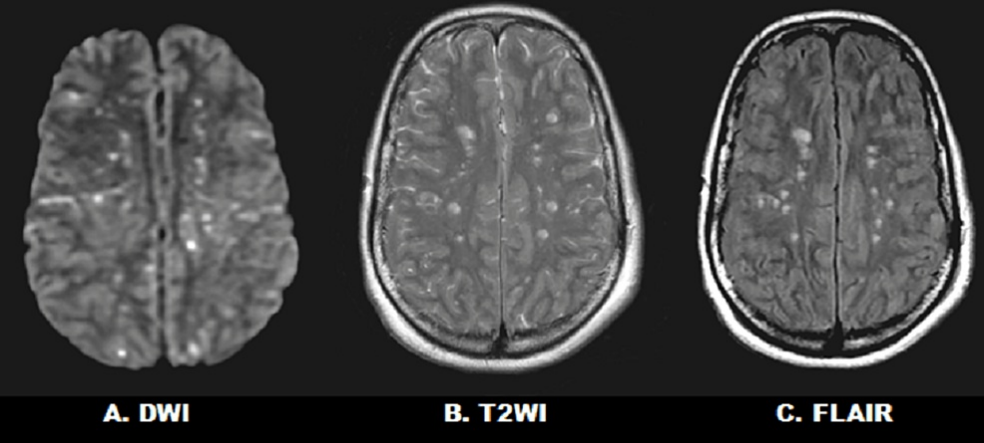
Case 2 with numerous cerebral white matter hyperintensities on (A) DWI, (B) T2WI, and (C) FLAIR MRI sequences within 48 hours of traumatic injury DWI, diffusion-weighted image; T2WI, T2-weighted image; FLAIR, fluid-attenuated inversion recovery; MRI, magnetic resonance imagining.
